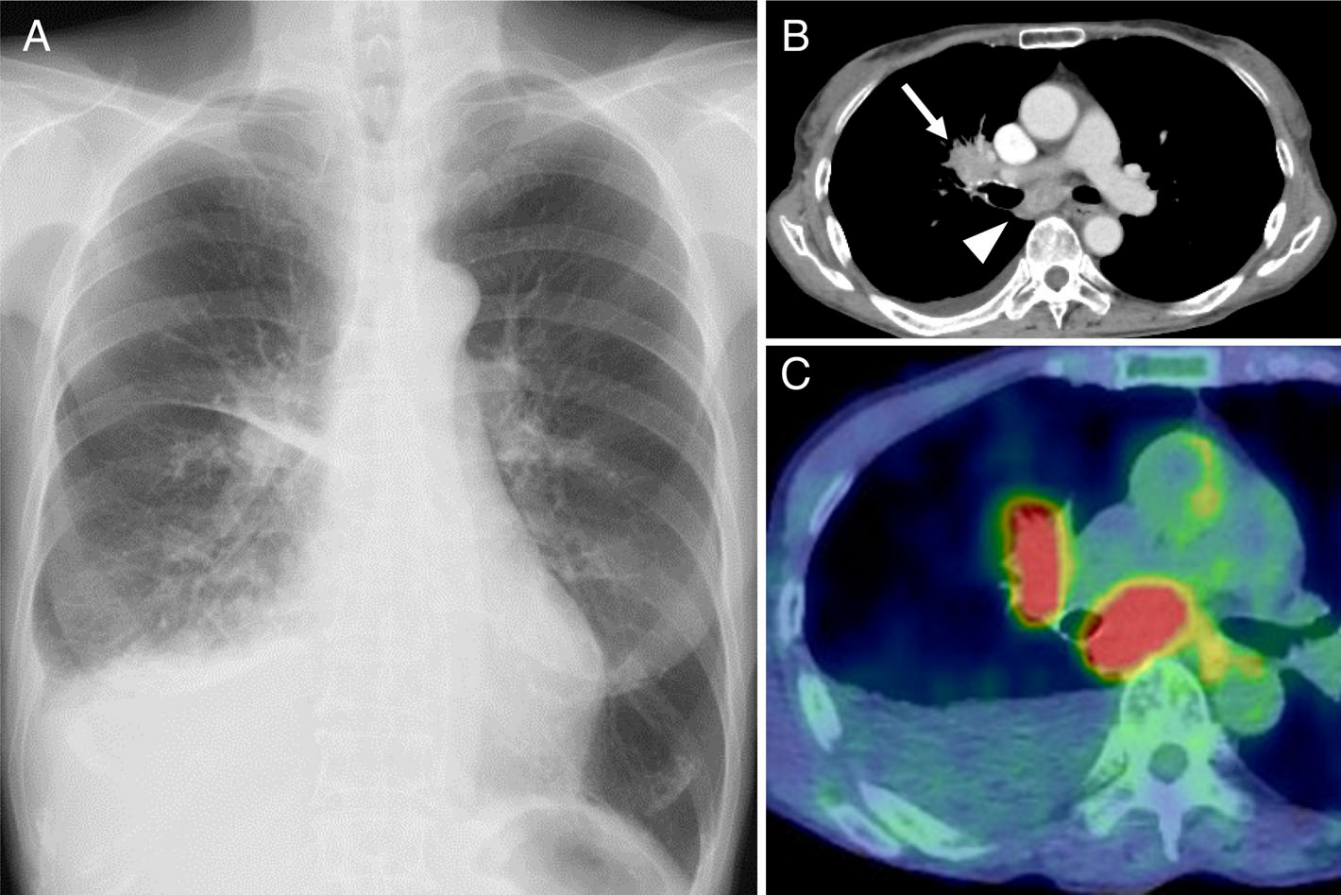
Chest X‐ray taken at the first visit depicted accumulation of moderate pleural effusion at the right side with thickening of the minor fissure together with right hilar lymphadenopathies (A). Thoracic computed tomography (CT) demonstrated a homogeneous enhanced mass measuring 3 cm in diameter (B, arrow) with subcarinal lymphadenopathies (C, arrow head). The fluorodeoxyglucose (FDG)–positron emission tomography (PET)–CT demonstrated a high FDG uptake in right hilar and subcarinal lymphadenopathies (C).
